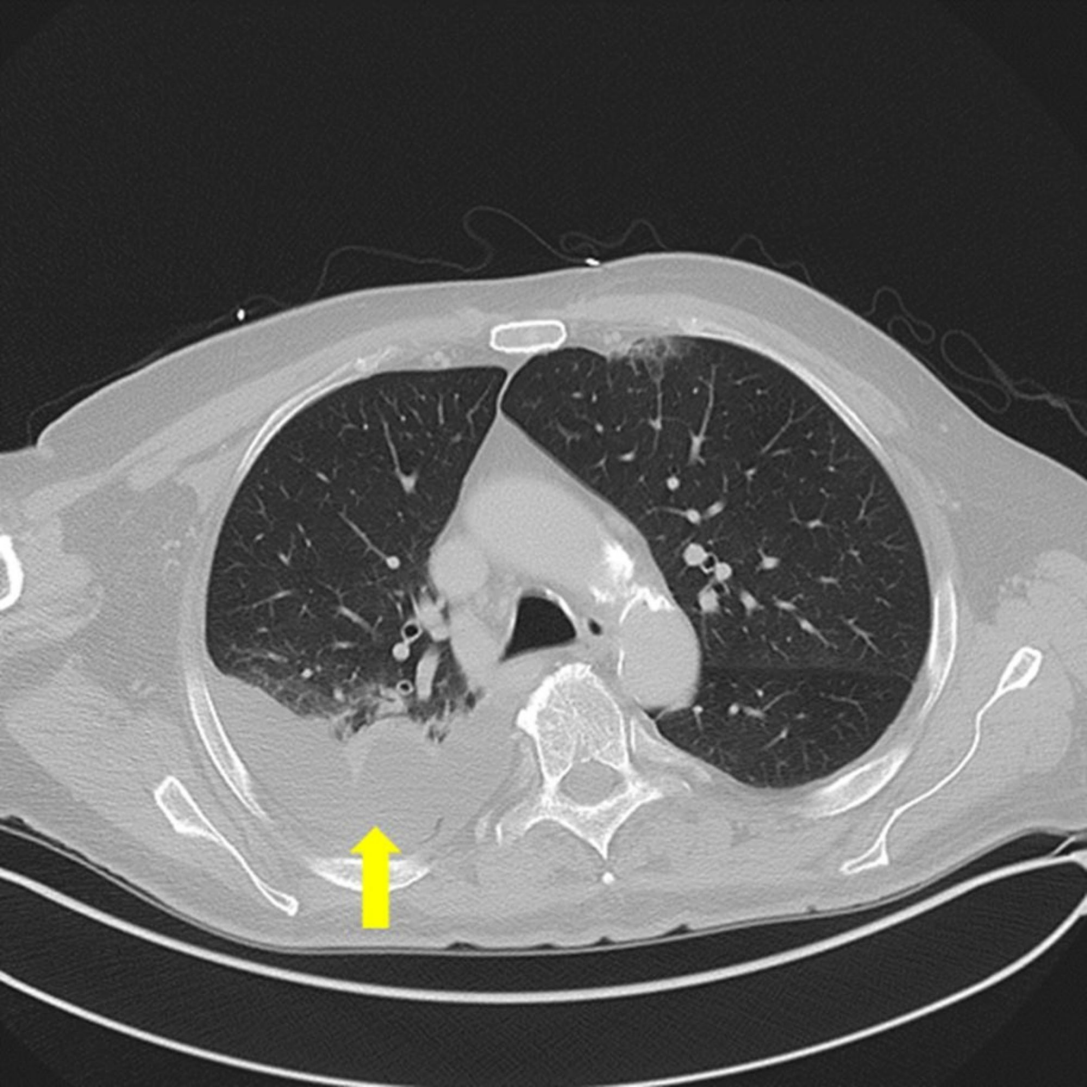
Chest computed tomography scan showing a right-sided basilar opacity with a moderate-sized pleural effusion (yellow arrow)
